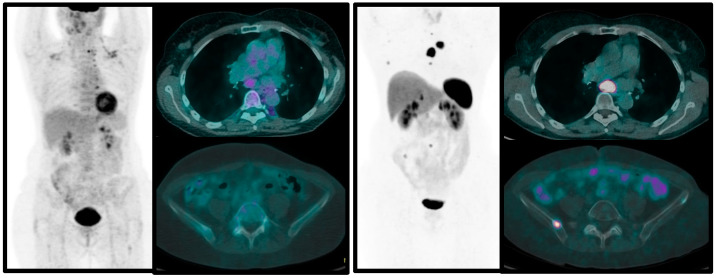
Fifty-eight-year-old female patient with previous history of TC of the inferior left lobe, treated with lobectomy, that 20 years after surgery developed multiple bone and nodal metastases (confirmed by biopsy). The patient underwent [^18^F]FDG (left) and ^68^Ga-DOTA-TOC PET/TC (right) within one week. All lesions show very high somatostatin receptors expression, but low glucose metabolism.
